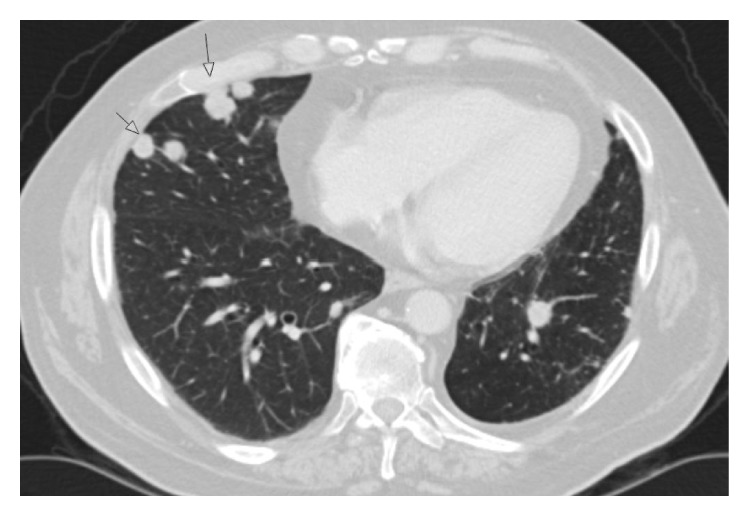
Computed tomography scan of the thorax showing progression of the right-sided pulmonary metastases approximately 3 months after radiotherapy to other targets. Temporary nontargeted effect.
